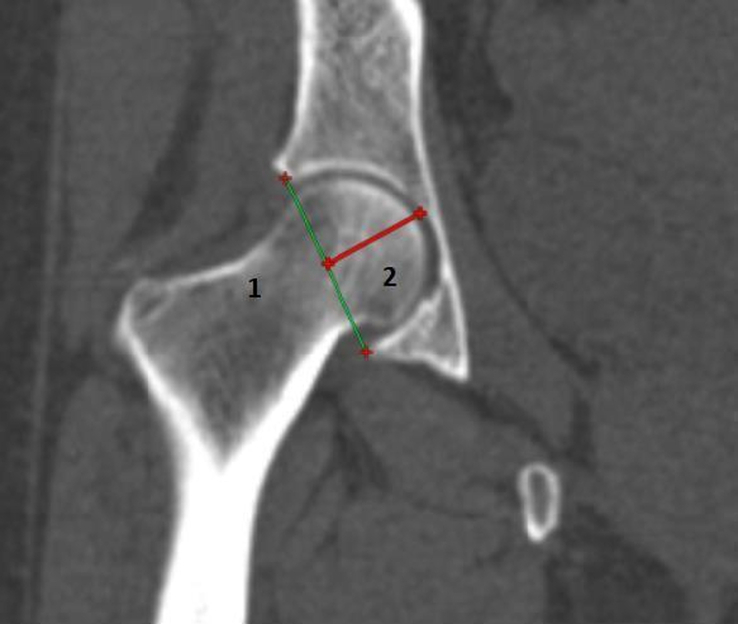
Reformatted coronal computed tomography image of right hip joint showing method for measurement of acetabular depth (Line 1: Line connecting superior and inferior acetabular margins, Line 2: Acetabular depth (perpendicular line drawn from midpoint of line 1 to dome of acetabulum).
